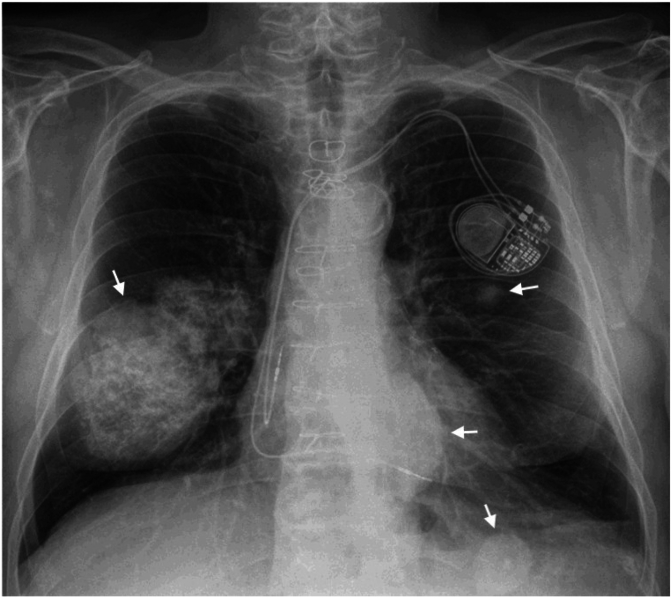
Chest radiograph revealing multiple calcified bilateral pulmonary nodules (arrows) of varying size.
